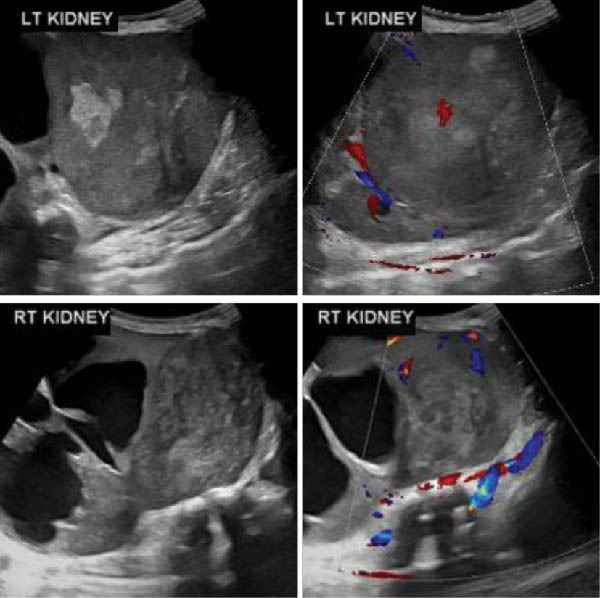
Renal ultrasound showing bilateral large lower pole renal masses crossing the midline. The masses show central hypoechoic/necrotic components and minimal internal vascularity. They are causing mass effect on the pelvicalceal system and subsequent hydronephrosis (Case 2).
